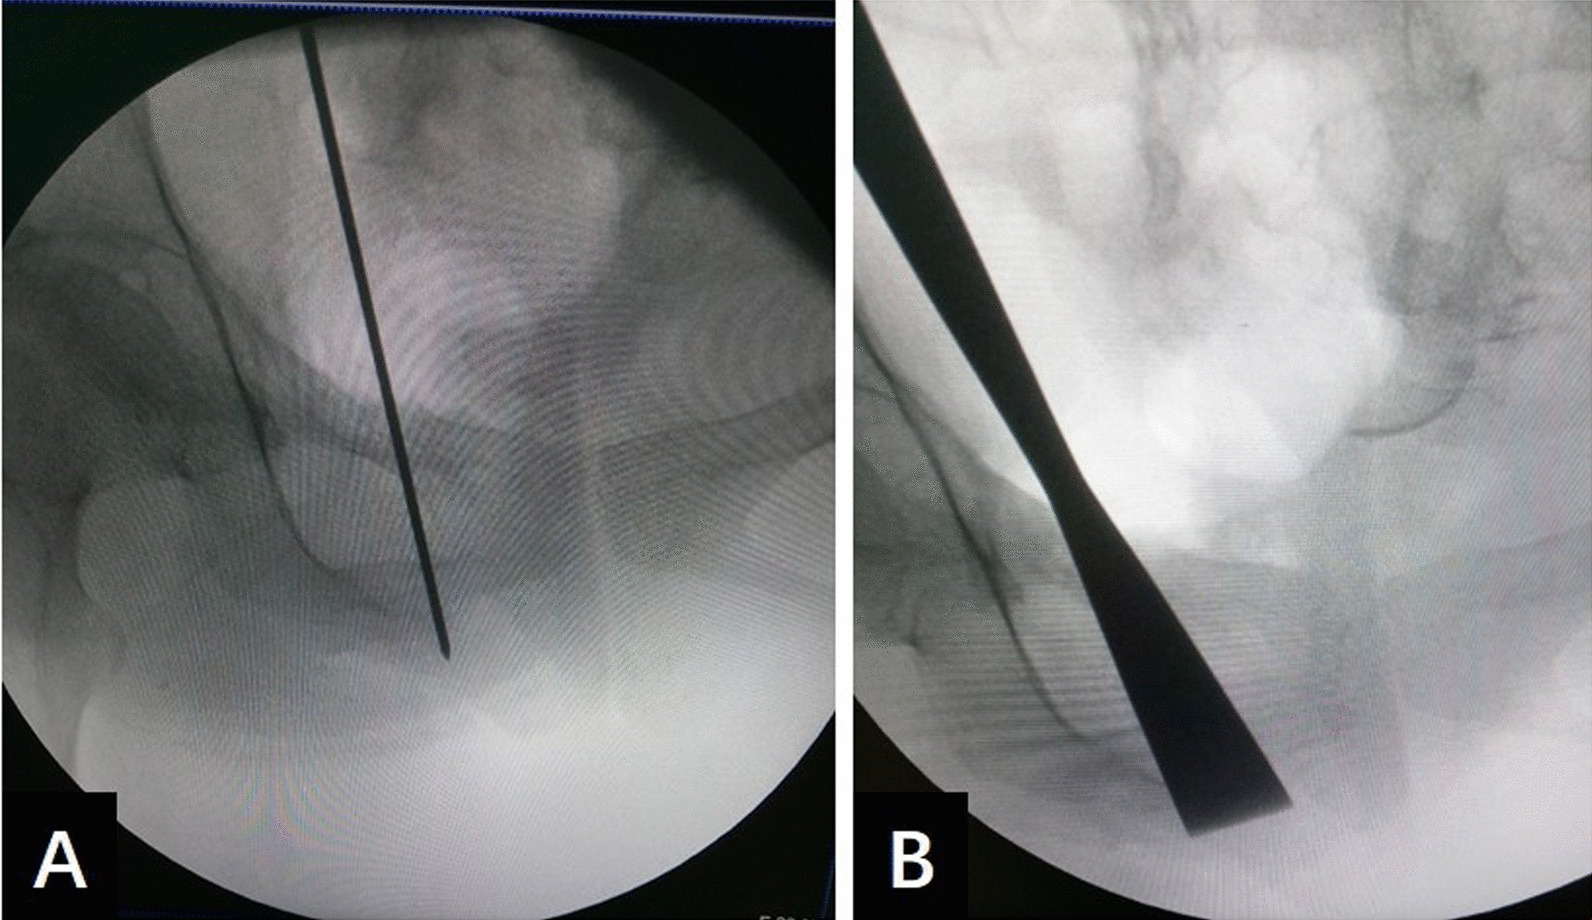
a Anteroposterior (AP) X-ray showing localization of the osteotomy line of the superior and inferior pubic rami with 2.0 K-wires. b Bone cut using an osteotome along the osteotomy line
